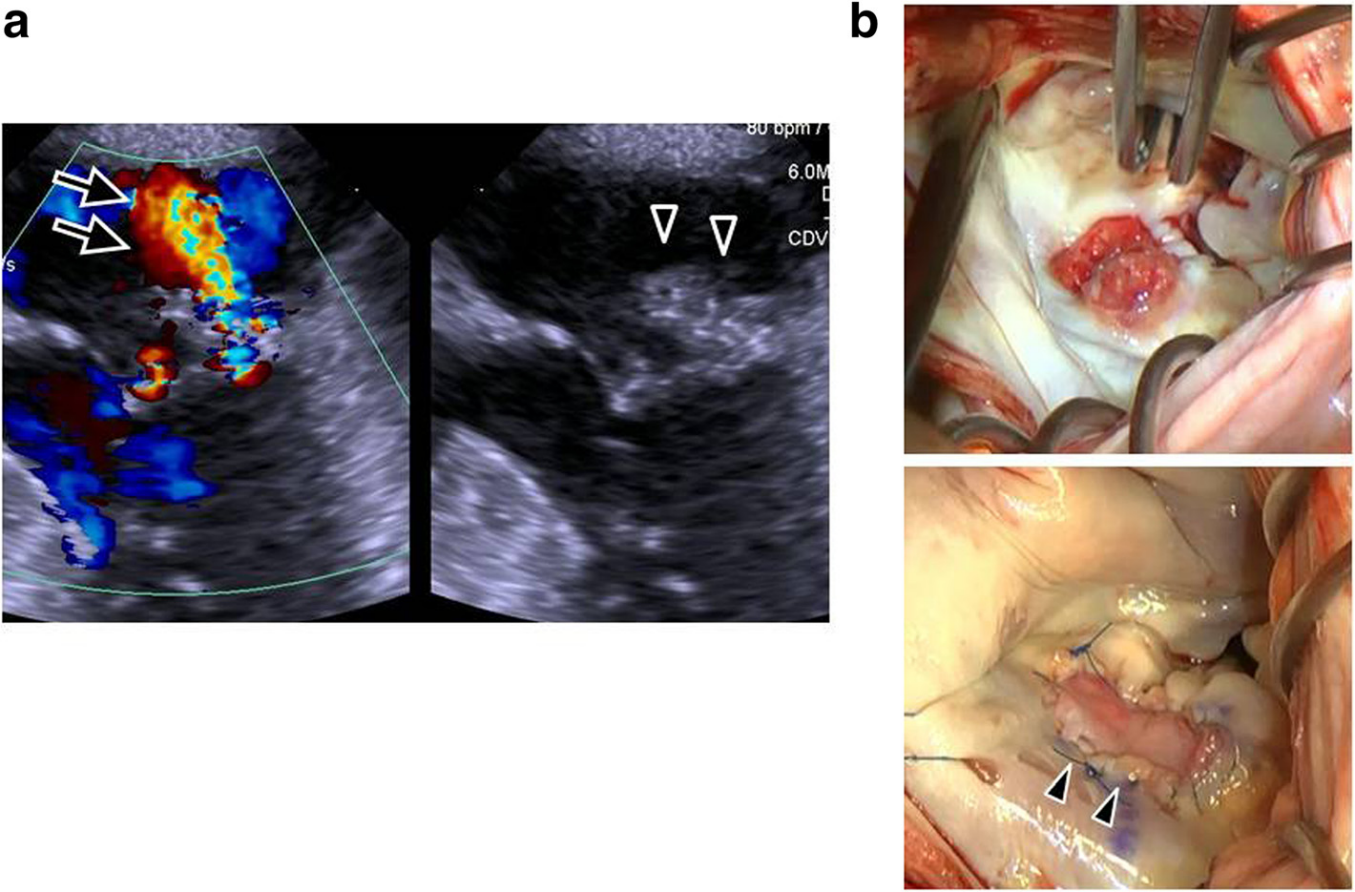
Concomitant mitral valve endocarditis. a Transesophageal echocardiography showed a large vegetation (15 × 17 mm) on the posterior mitral leaflet (*arrowheads*) and moderate insufficiency because of leaflet perforation (*arrows*). b Intraoperative mitral valve findings through the left atrial approach. *Upper*; A large vegetation was present on the middle posterior leaflet {P2 scallop}. *Lower*; Complete resection and repair of mitral valve using autologous pericardial patch (*arrowheads*). Concomitant mitral valve endocarditic
